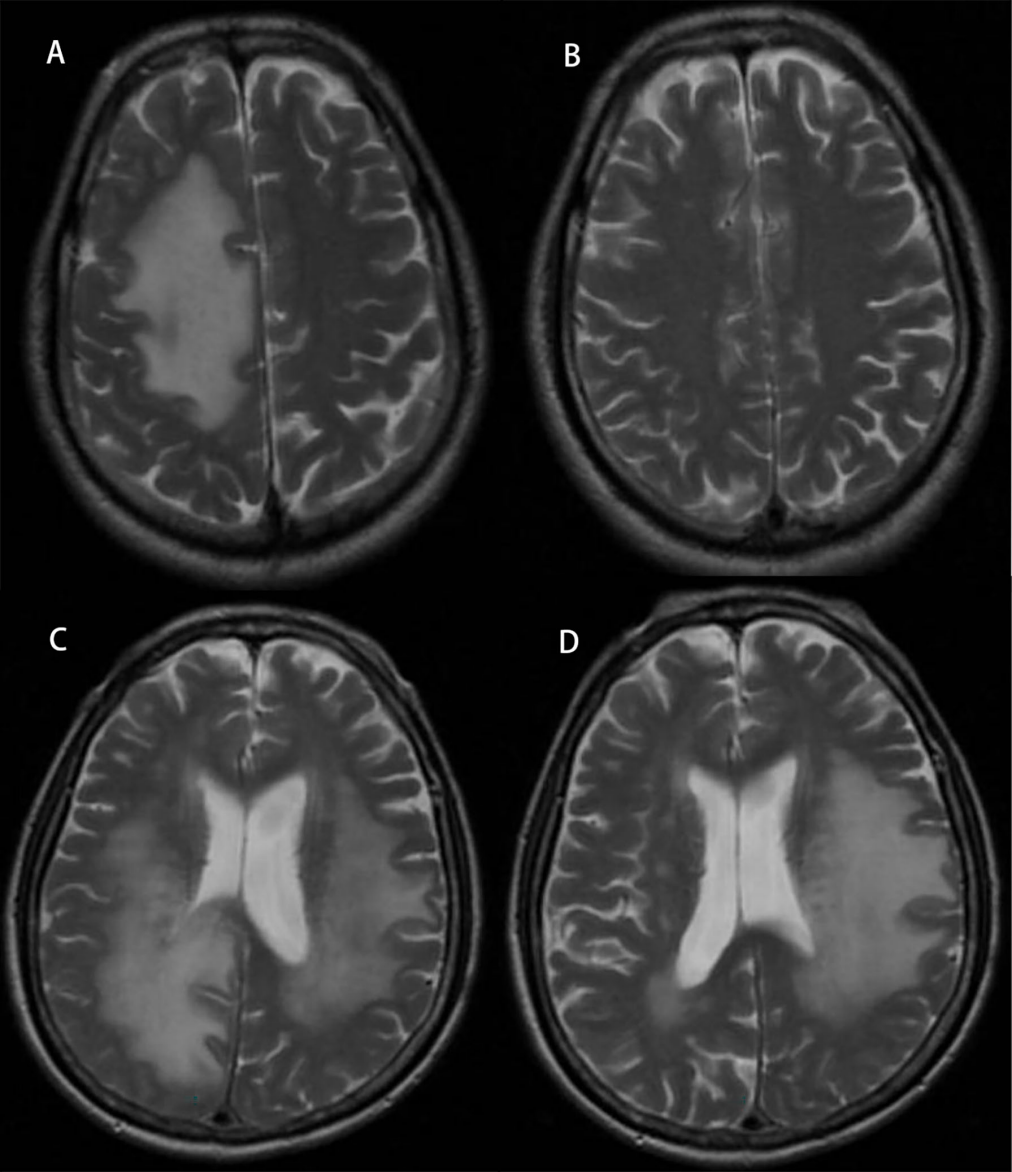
Radiographic images of brain edema before and after treatment with bevacizumab. Panel (A) represents edema in a lung cancer patient before treatment, and panel (B) represents after treatment. Panel (C) represents edema in a colon cancer patient before treatment, and panel (D) represents after treatment.
